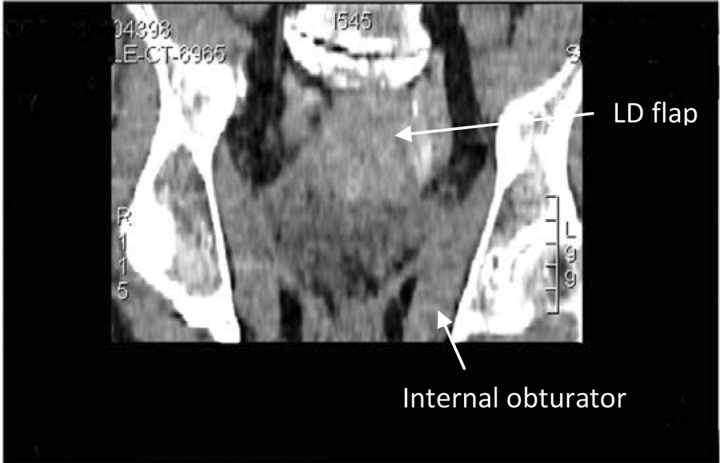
Coronal computed tomography of the pelvis following latissimus dorsi (LD) reconstruction of perineum and pelvis
